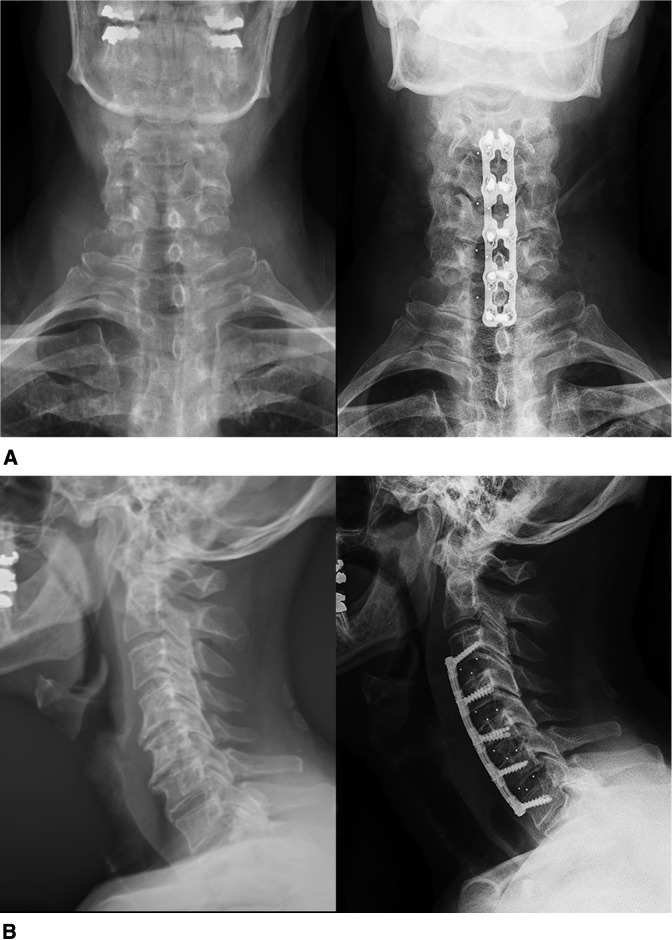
Radiographs of the cervical spine showing (A) preoperative (left) and postoperative (right) AP views and (B) preoperative (left) and postoperative (right) lateral view.
